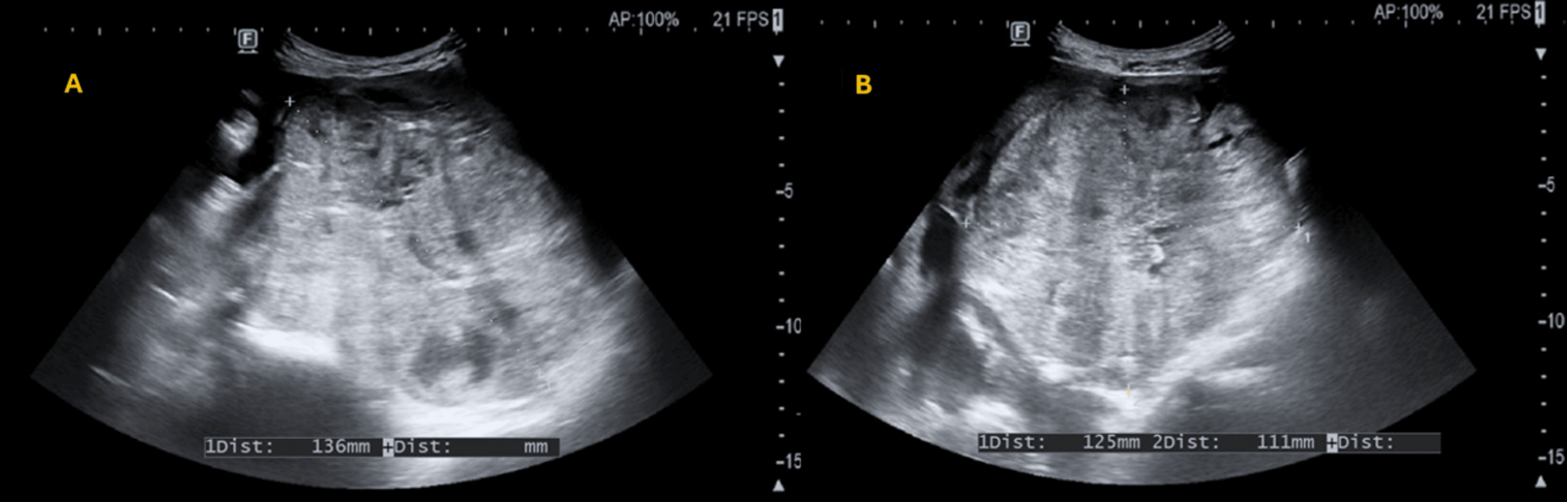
Abdominopelvic ultrasound of biplanar pelvic mass measurements: panels A and B. Panel A (sagittal, long-axis view) shows a well-circumscribed mass occupying the pelvis, measuring 136 mm in the craniocaudal dimension (blue callipers). Panel B (axial, transverse view) shows the same lesion in cross-section, measuring 125 mm in the transverse (left-to-right) axis and 111 mm in the anteroposterior axis. The lesion exhibits mixed echotexture and causes displacement of adjacent pelvic structures, consistent with a space-occupying pelvic mass.
